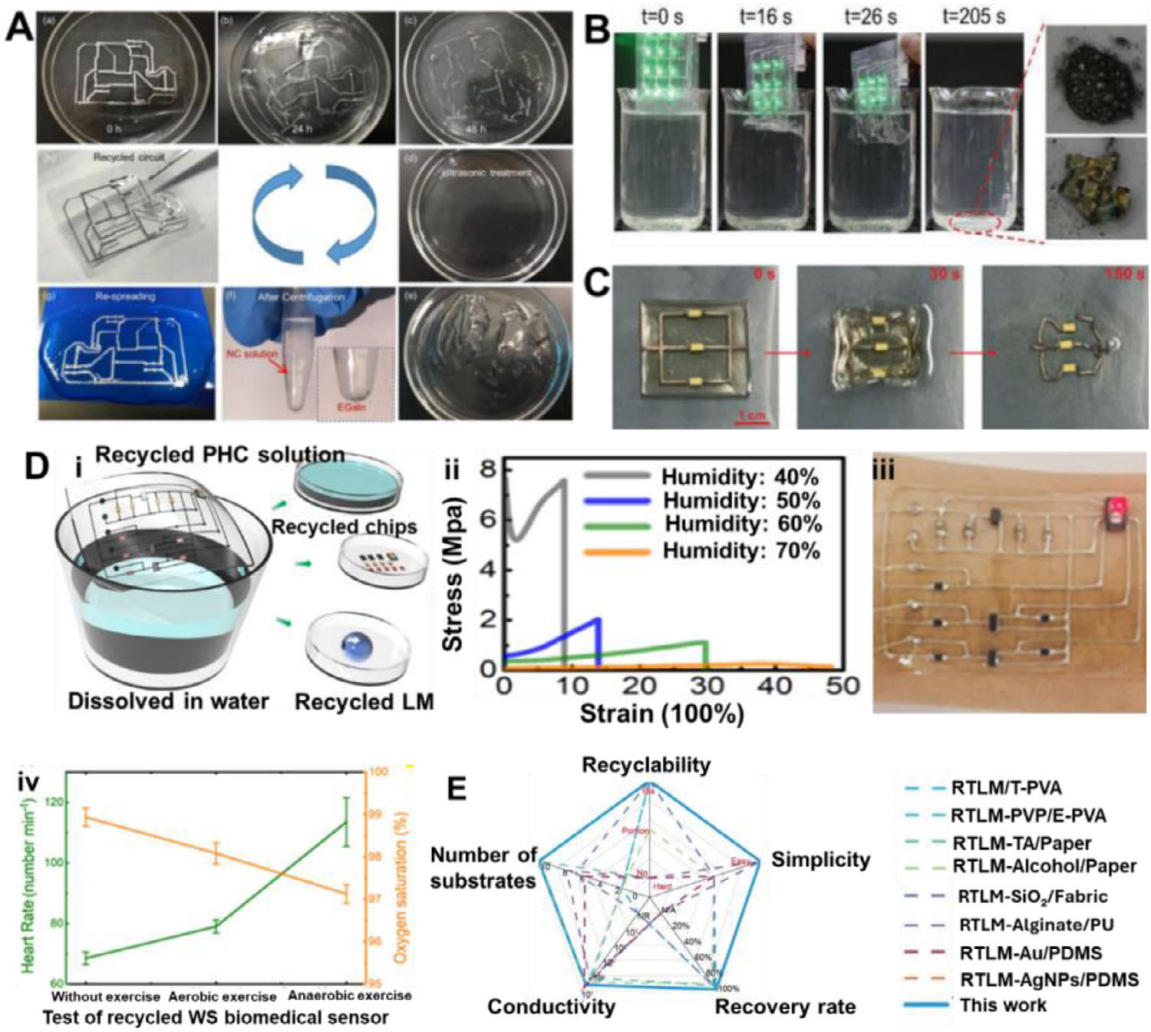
A) Dissolution and reuse of the NC‐LM circuit: i) immersion in water; ii) fragmentation after 24 h; iii) blurred LM pattern after 48 h; iv) ultrasonic‐assisted degradation; v) uniform NC‐LM mixture; vi) LM and NC separated by centrifugation; vii) NC re‐applied; viii) recycled circuit with original morphology. Reproduced with permission,^[^
